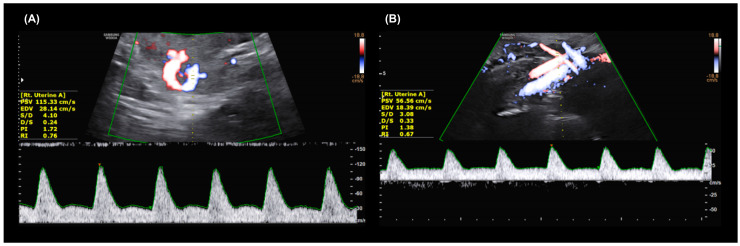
Representative transabdominal Doppler images of the uterine artery (A) in the first trimester (10–13 weeks) with an early diastolic notch and (B) in the second trimester (20–26 weeks) with normal uterine artery waveforms.
